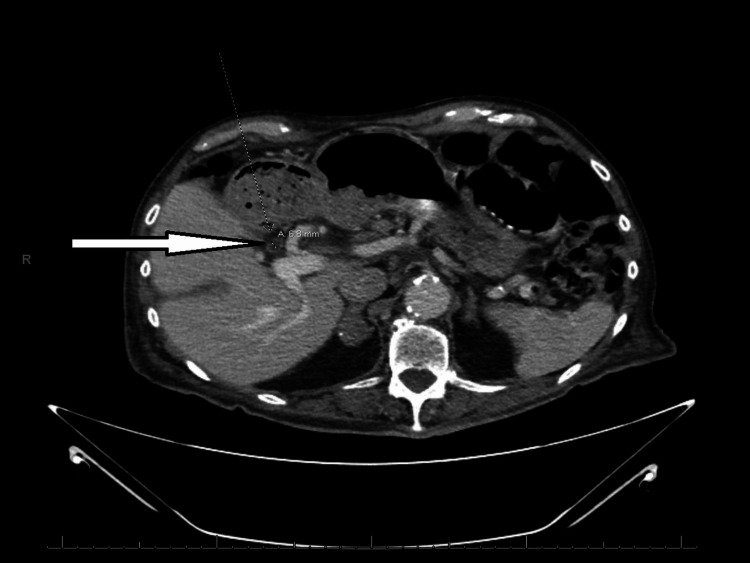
CT abdomen and pelvis on presentation showing thickening duct wall (arrow), which is non-specific but could represent cholangitis in the correct clinical setting.
